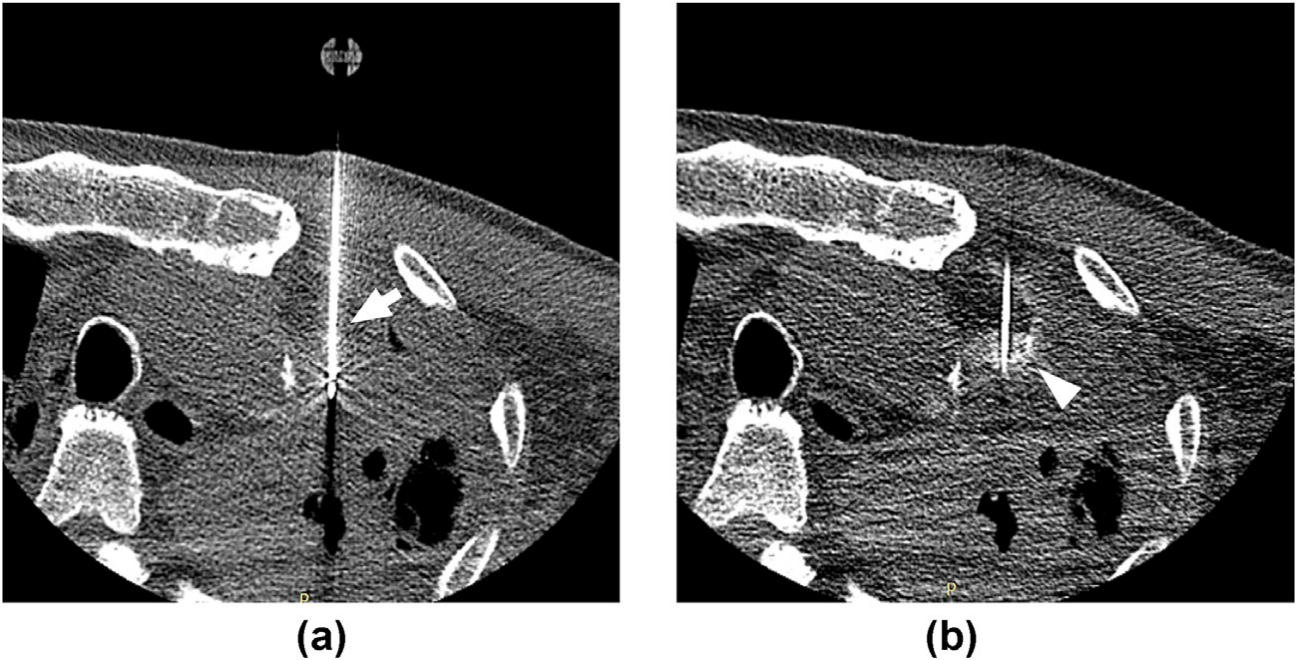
CT images during the RFA procedure. The tumor is punctured from the anterior chest wall with a Cool-Tip 2-cm electrode (A, arrow). Injected xylocaine mixed with contrast medium is seen as high density area around the tumor. The xylocaine mixture remains in the anterior aspect of the mass (arrowhead), which could not be separated from the aorta (B).
